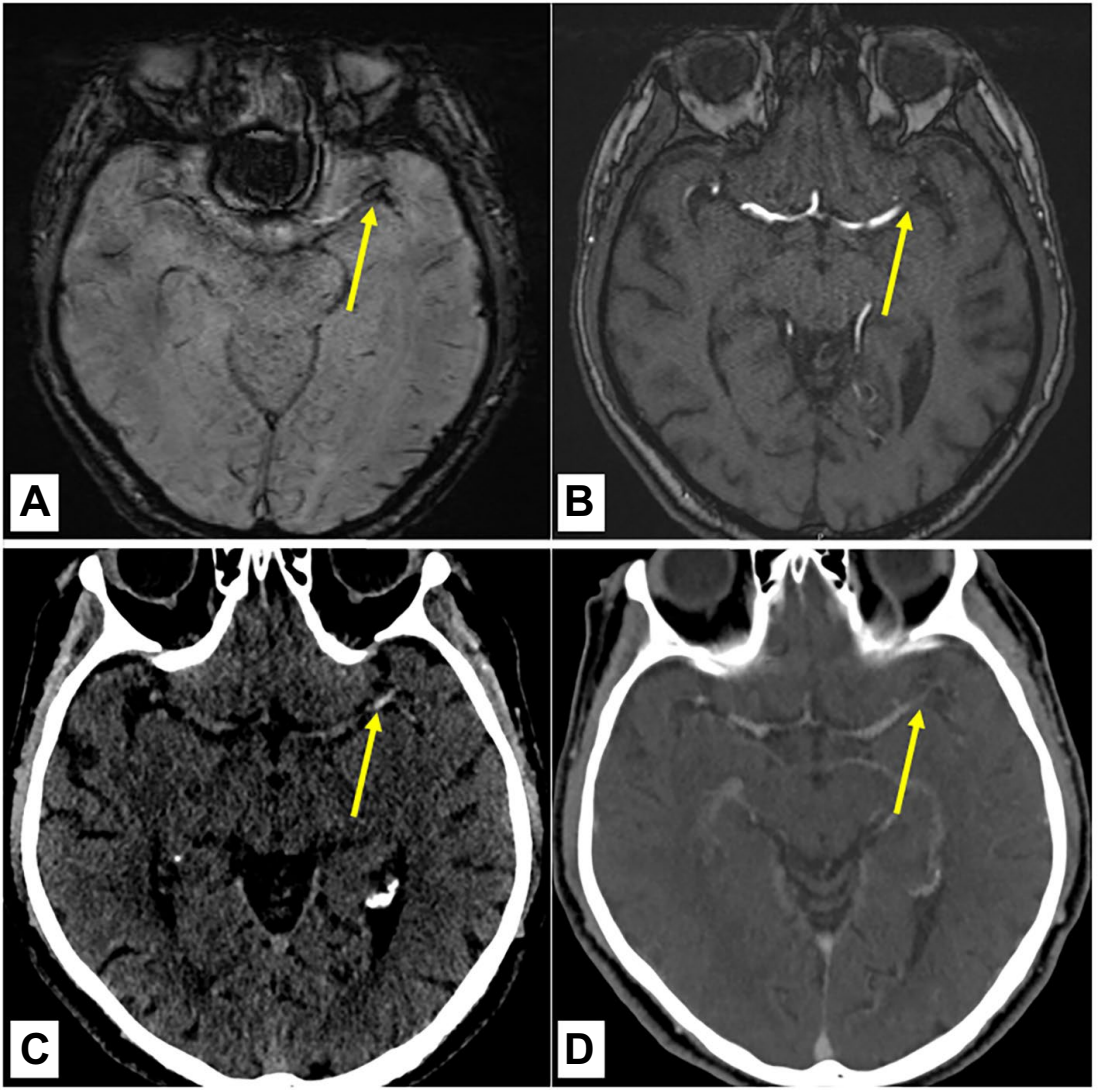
Assessment of the susceptibility vessel sign and hyperdense vessel sign status on baseline brain imaging. (A,B) 70-year-old male patient with an acute ischemic stroke due to the occlusion of the left MCA (M1 segment) diagnosed on baseline brain MRI with present SVS (arrowhead) as local hypointensity on the SWI (A) with confirmation of occlusion on the arterial TOF (B). (C,D) Externally performed CT imaging of the same patient before referring for thrombectomy. Presence of HVS (arrowhead) as local hyperdensity in native CT (C) with confirmation of occlusion in the CT angiography (D). Yellow arrows point to the proximal part of the vessel occlusion. HVS indicates hyperdense vessel sign; MCA, middle cerebral artery; SVS, susceptibility vessel sign; SWI, susceptibility weighted imaging and TOF, time of flight angiography.
